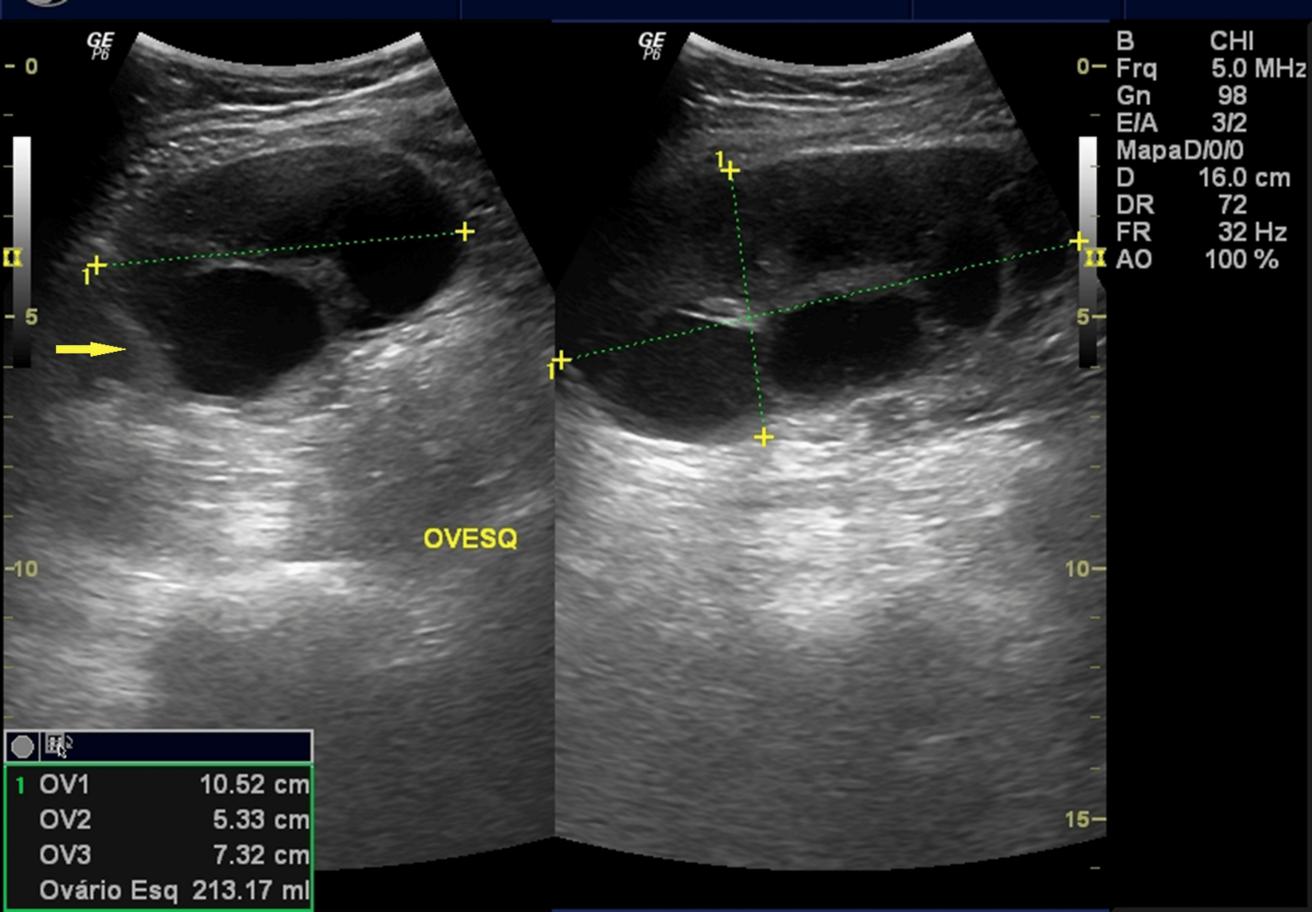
Left ovary with theca lutein cysts. Transvaginal ultrasound demonstrating an enlarged left ovary containing multiple theca-lutein cysts. The arrow indicates a representative cyst.
